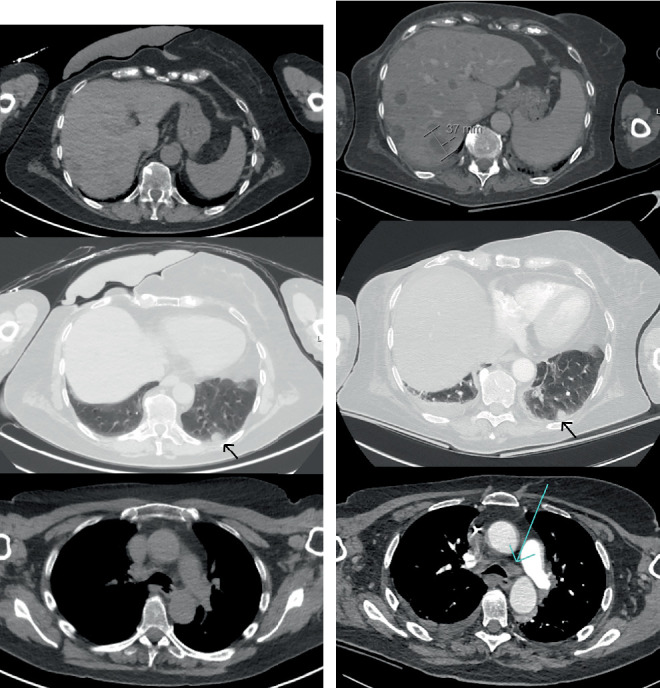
(a) CT images from FDG-PET/CT in August 2024. (b) CT during admission in November 2024; multiple liver metastases are visible, with the largest lesion measuring 37 mm. A stable lung metastasis is indicated by the black arrow, and a new mediastinal lymphadenopathy is seen in Region 4L, marked by the blue arrow.
